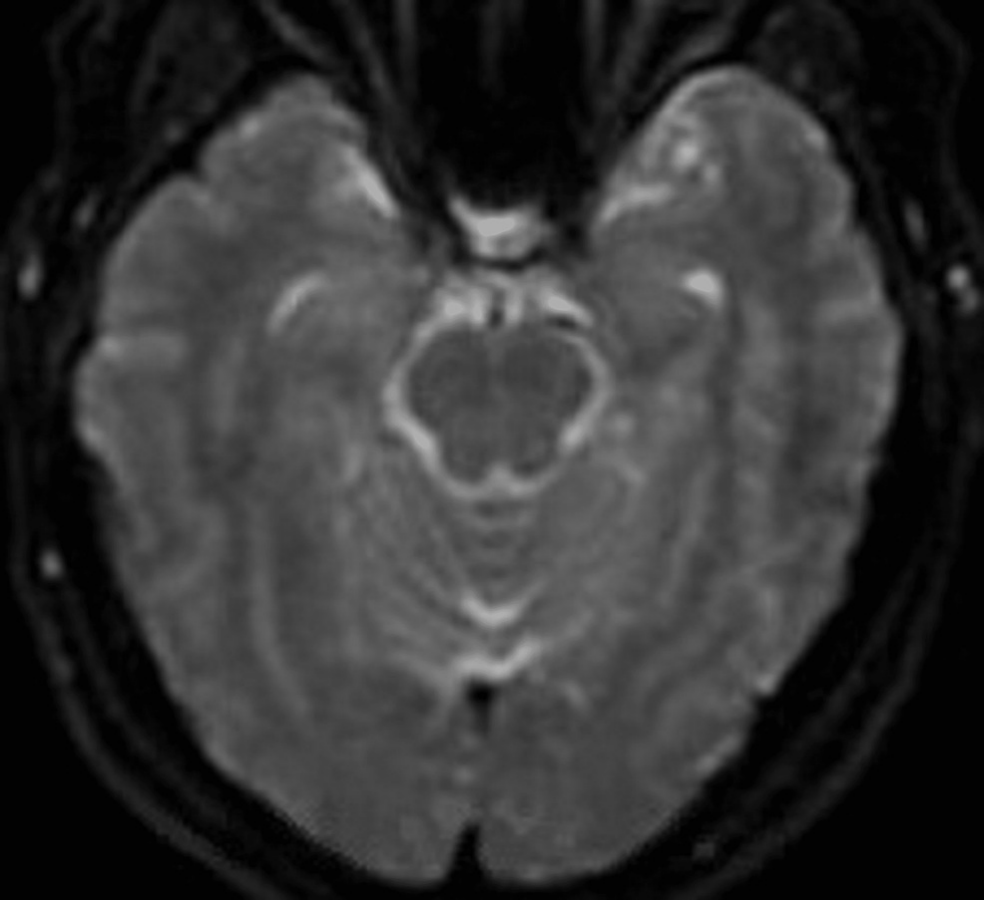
MRI T2-weighted sequence 13 hours after the episode
